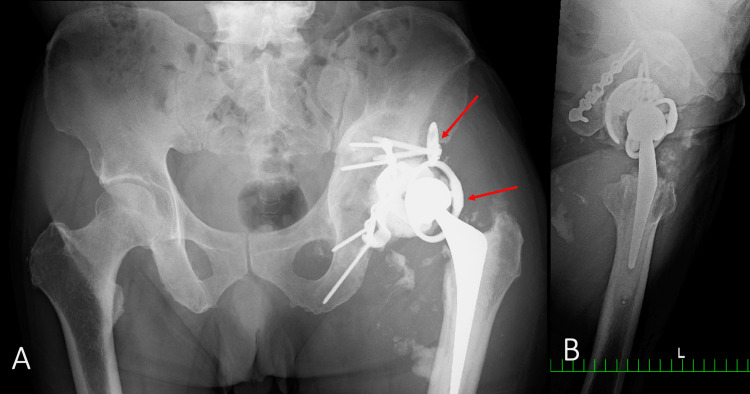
Plain radiograph one year after the second surgery A) Frontal radiograph; B) Lateral radiograph Displacement of the acetabular cup and dislocation of screws in the reconstruction plate are revealed (red arrows
